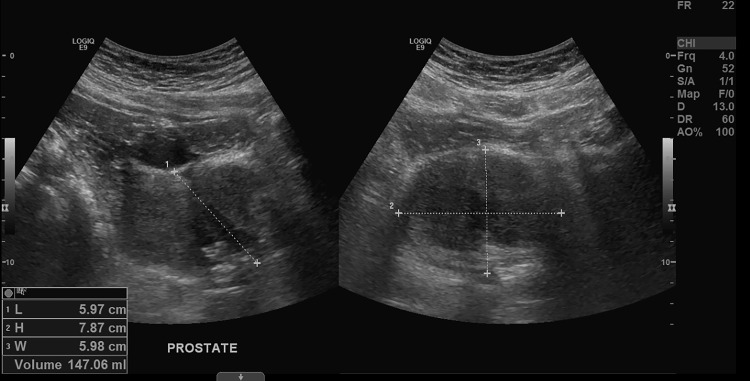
Retroperitoneal ultrasound Retroperitoneal ultrasound demonstrated marked prostatic gland enlargement (7.9 cm × 5.9 cm × 5.9 cm) with median lobe hypertrophy.
